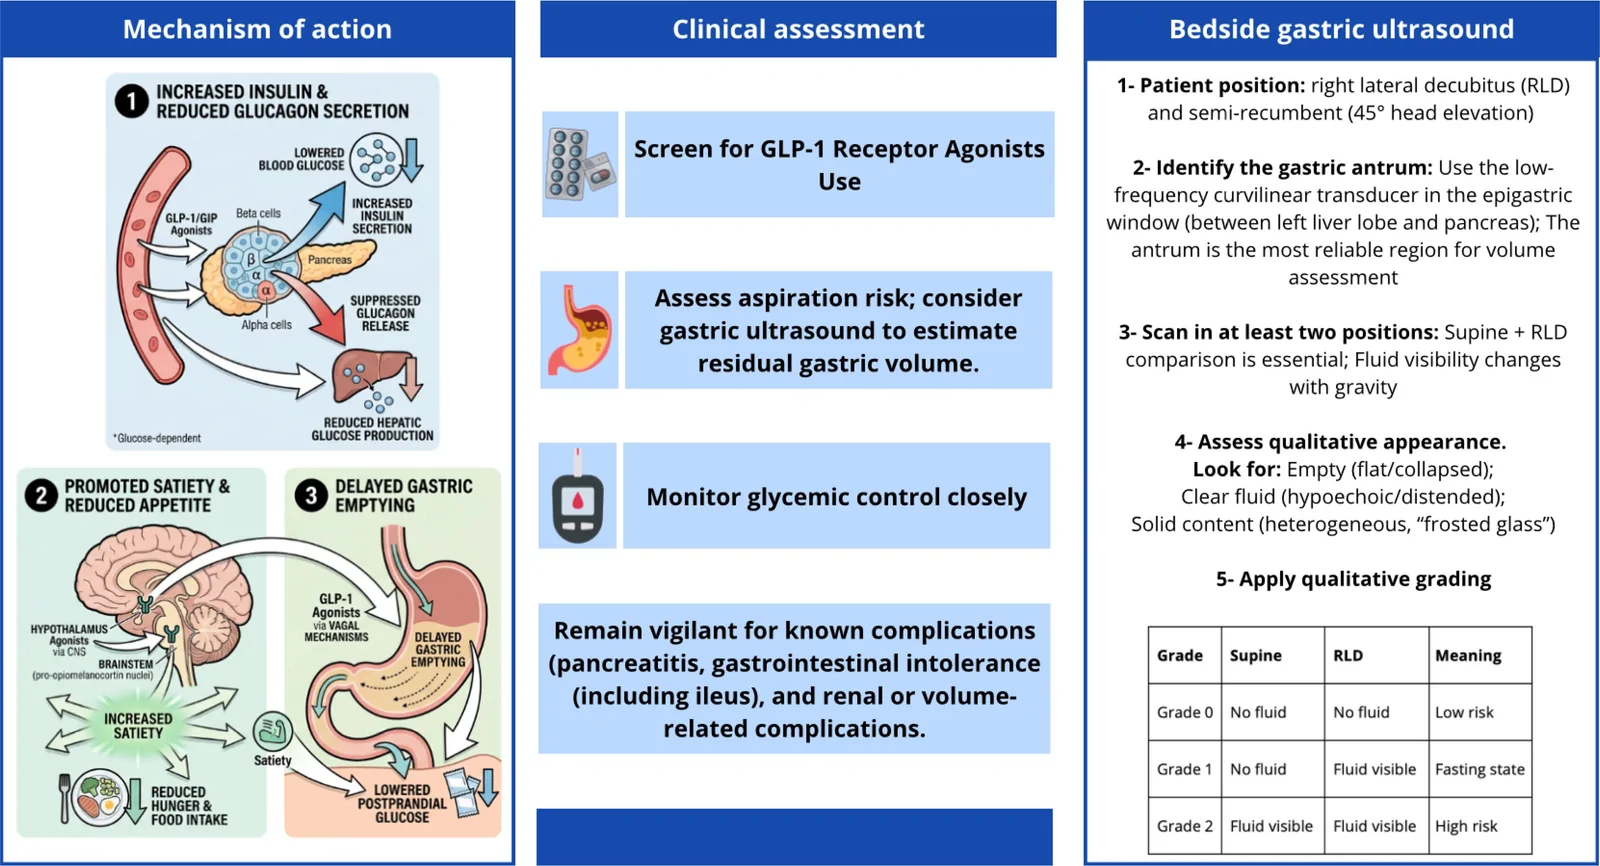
Overview of glucagon-like peptide-1; glucose-dependent insulinotropic polypeptide receptor agonists in critical care: mechanisms of action, a stepwise clinical assessment approach, and key elements of bedside gastric ultrasound for aspiration risk evaluation.^(4)^
